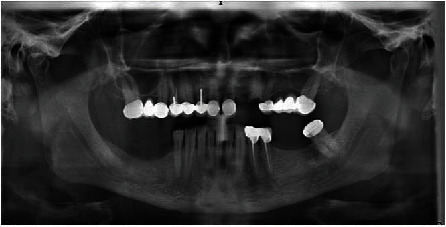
Patient's orthopantomograph.
